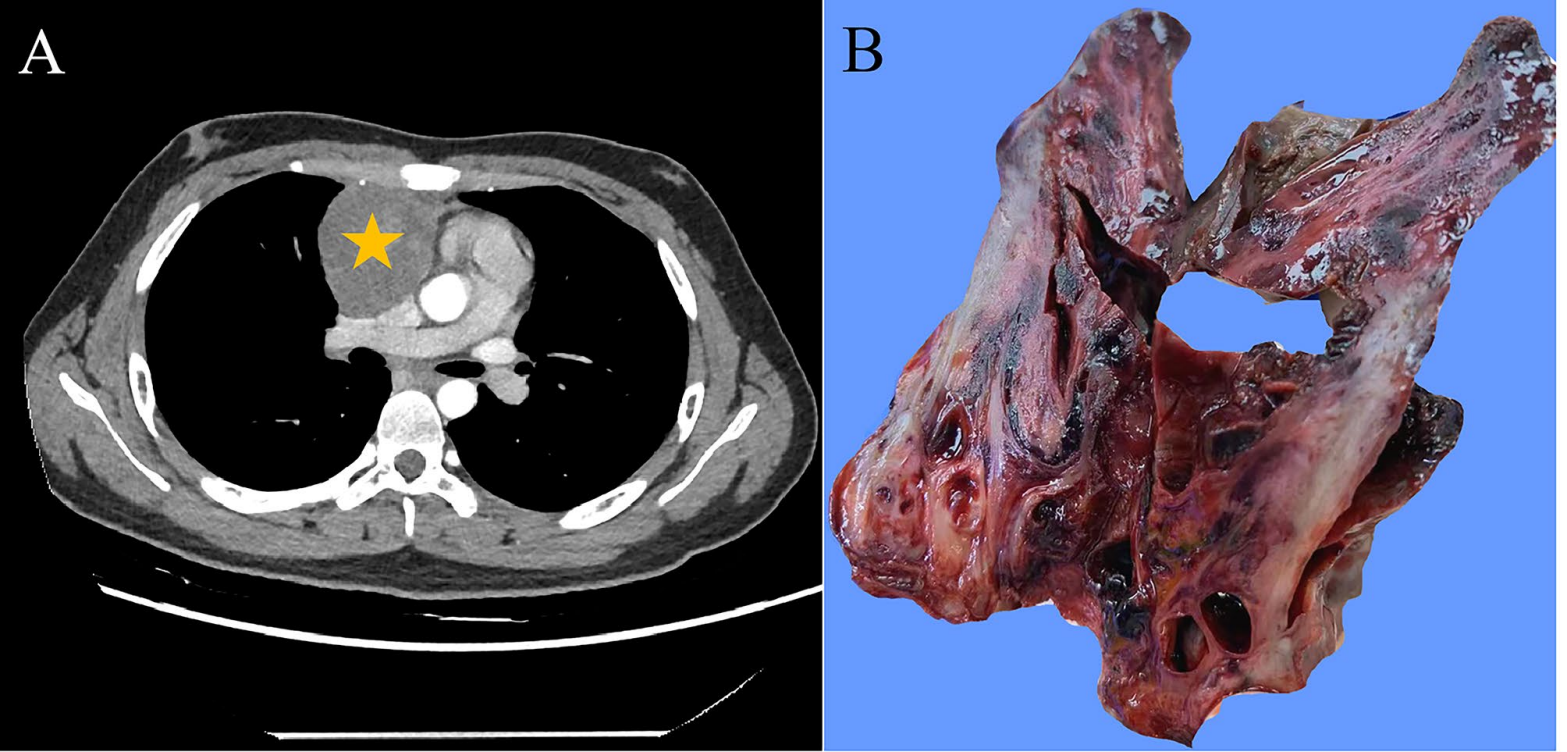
Imaging and gross examination of the mediastinal tumour. (A) CT demonstrates a solid mass (yellow star) in the right anterior mediastinum with uneven density and well-defined boundaries. (B) The cut surface of the tumour was greyish‒white solid mixed with haemorrhagic cystic changes
